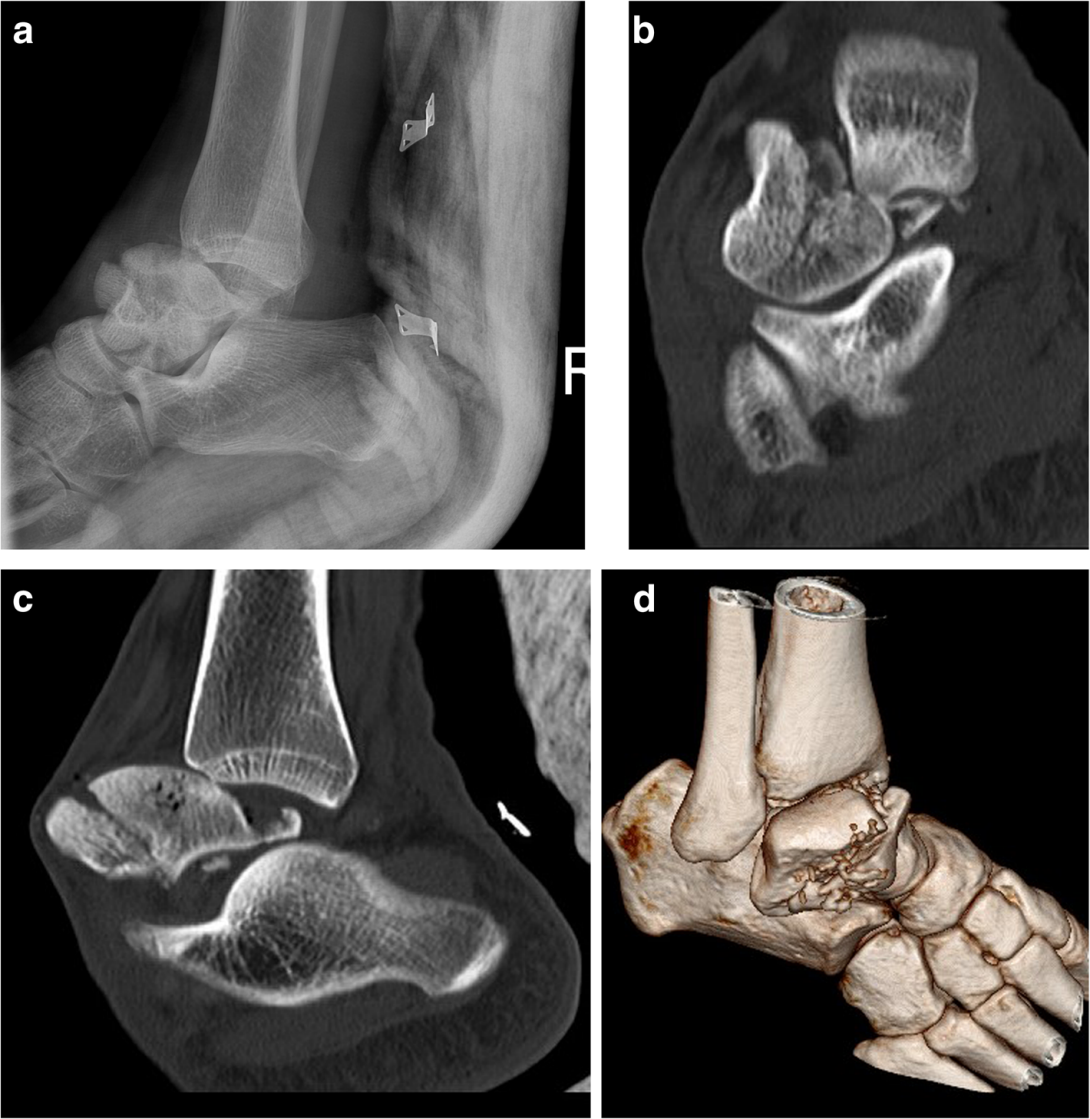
Pantalar fracture dislocation with comminuted fracture of head & neck in a 26-year-old man. Radiograph (a), CT sections (b-d)
